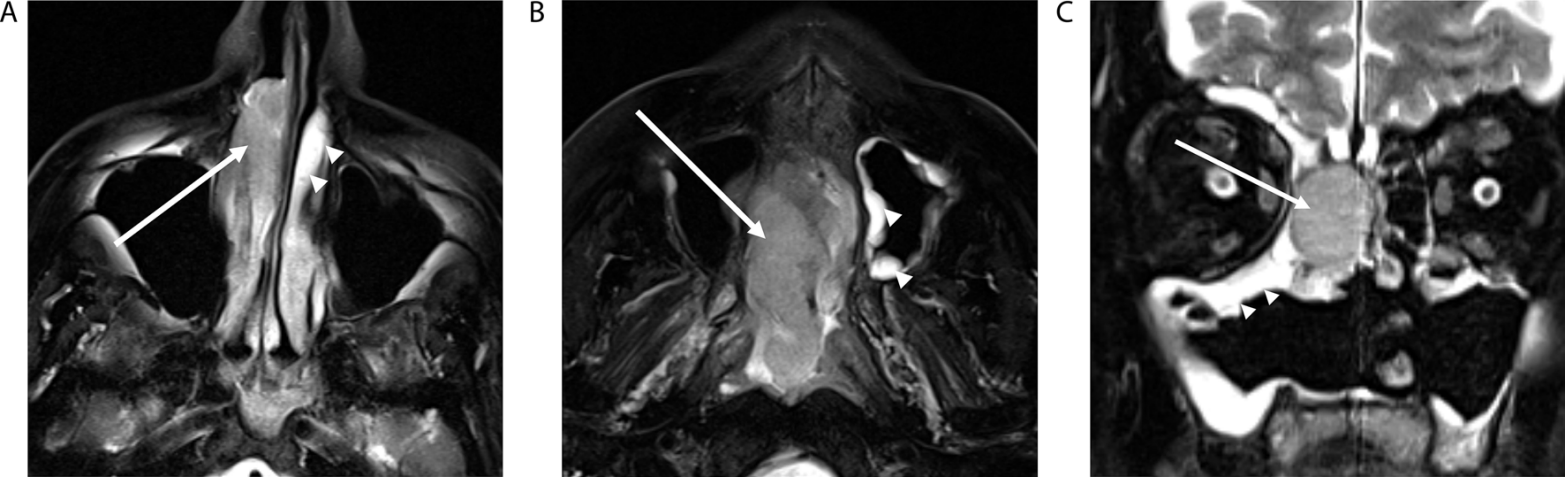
Axial T2-weighted fat-suppressed (A and B) and coronal T2-weighted fat-suppressed (C) MR images in a patient with multiple-recurrent sinonasal mucosal melanoma with identical intermediate T2 signal intensity of the tumor (arrows) compared to the T2 hyperintense signal in the normal mucosa (arrowheads) at initial staging (A), NI-RADS MRI primary category 3 first recurrence (B), and NI-RADS primary category 3 second recurrence (C)
